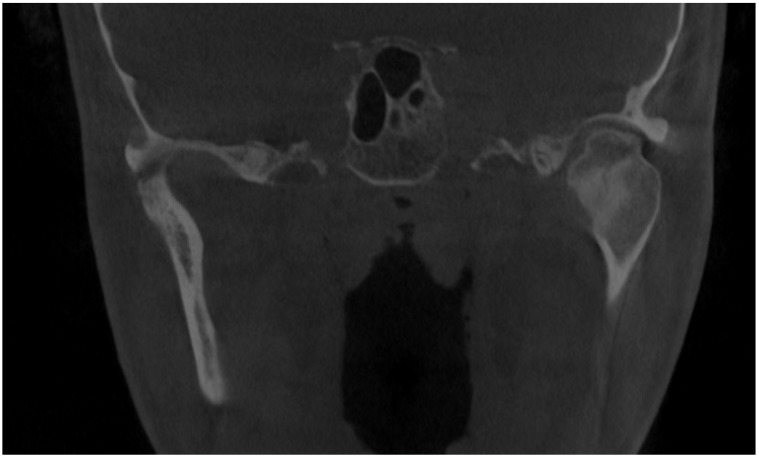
Initial scan—CBCT, coronal view—enlargement and overgrowth of left condylar head causing visible mandibular and skeletal asymmetry. The patient underwent orthodontic treatment (2006–2010) and scheduled BSSO (bilateral sagittal split osteotomy) for surgical correction of the asymmetrical mandibular and featured dentofacial deformity. Six years after the procedure, the patient demonstrated signs of re-occurrence of mandibular asymmetry. After some corrective orthodontic approaches, consultations, and treatment proposals, the patient was scheduled for consultation in our ward (2021–2022). Because of severe mandibular asymmetry, unilateral open bite, chin deviation, midline shift towards the healthy right side, enlargement and overgrowth of the left mandibular basis and ramus, a suspicion of condylar hyperplasia was raised [1,2]. Because the CBCT scans revealed overall extensive progressive growth of the left condylar head, a decision for additional SPECT was decided. According to the known literature, a one-sided open bite with chin deviation towards the heathy opposite side, followed by mandibular corpus enlargement and elongation, are quite common clinical syndromes of condylar hyperplasia. The scope of visible changes in mandibular anatomy is greatly dependent on the time of this abnormal pathological growth and its intensity. It is quite important to evaluate each patient individually because mandibular abnormal growth might not only lead to dentoalveolar changes but also skeletal changes, which, depending on their intensity, might require some degree of surgical intervention. Rarely, condylar hyperplasia might present itself as an osteochondroma or other rare temporomandibular joint tumors [1,2,3]. Therefore, each case of asymmetry, bone change, visible bone enlargement, and overgrowth should be carefully evaluated. Abbreviations: CBCT—cone beam computed tomography, SPECT—single-photon emission computed tomography.
